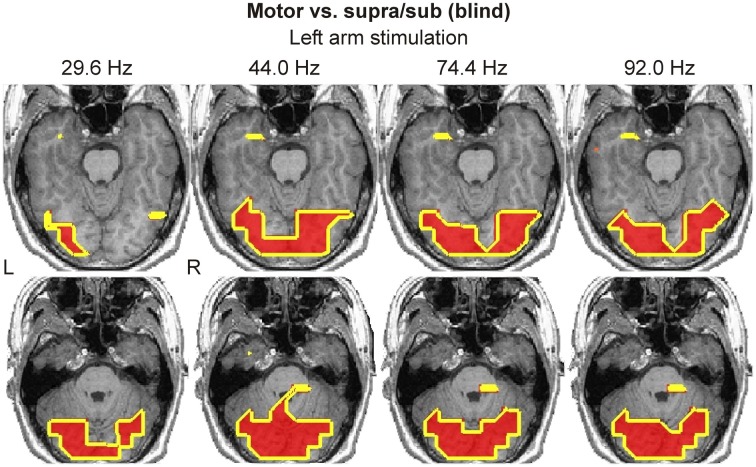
Spatio-frequency activation maps of the blind subject derived from “Motor vs. Supra/Sub” contrast are shown for left arm stimulation at four frequencies (indicated on top of each column). Two axial slices of MRI best covering the visual cortex activations are shown. Yellow contours encompass regions with statistically significant (*P* < 0.0001) increases in activity.
